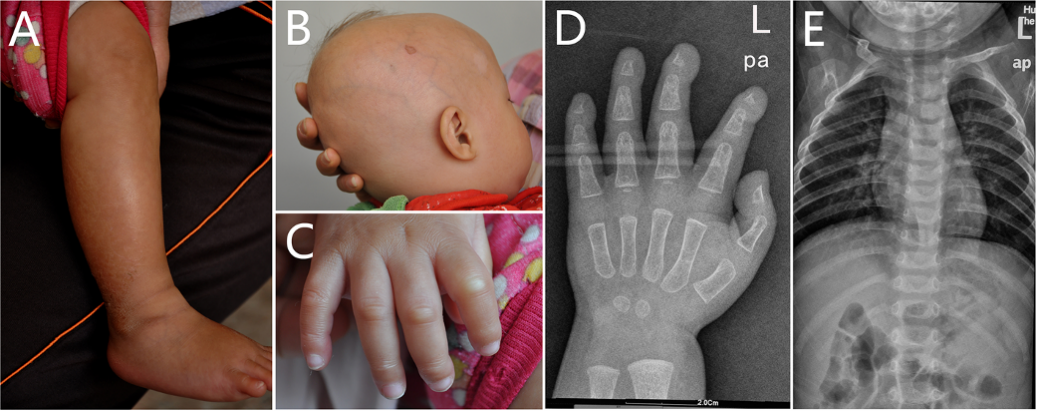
Patient 3 shows swelling with mild hyperpigmentation on lower limb (A), decreased scalp hair with prominent scalp vein (B) and mild contractures at the interphalangeal joints of hands (C). X-ray findings show acro-osteolysis of distal phalanges, normal clavicles and ribs (D, E).
